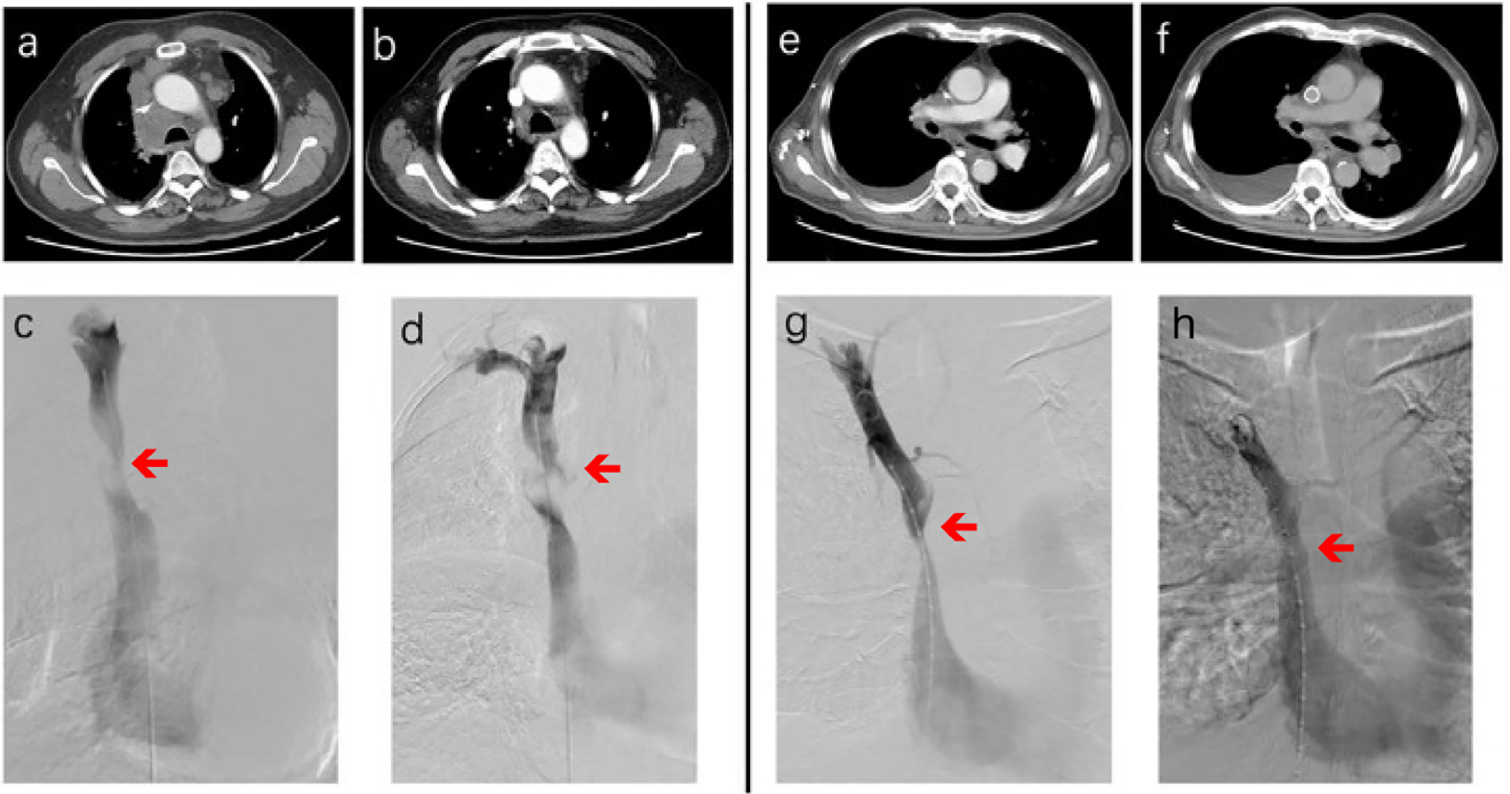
The pre‐ and postoperative images of the two patients with superior vena cava syndrome (SVCS). (a–d) Patient 1 and (e–f) patient 2. (a and e) Preoperative computed tomography (CT) scan images of both patients; and (b and f) postoperative CT scan images of both patients. (c and g) Preoperative angiography of both cases; and (g and h) postoperative angiography of both cases.
